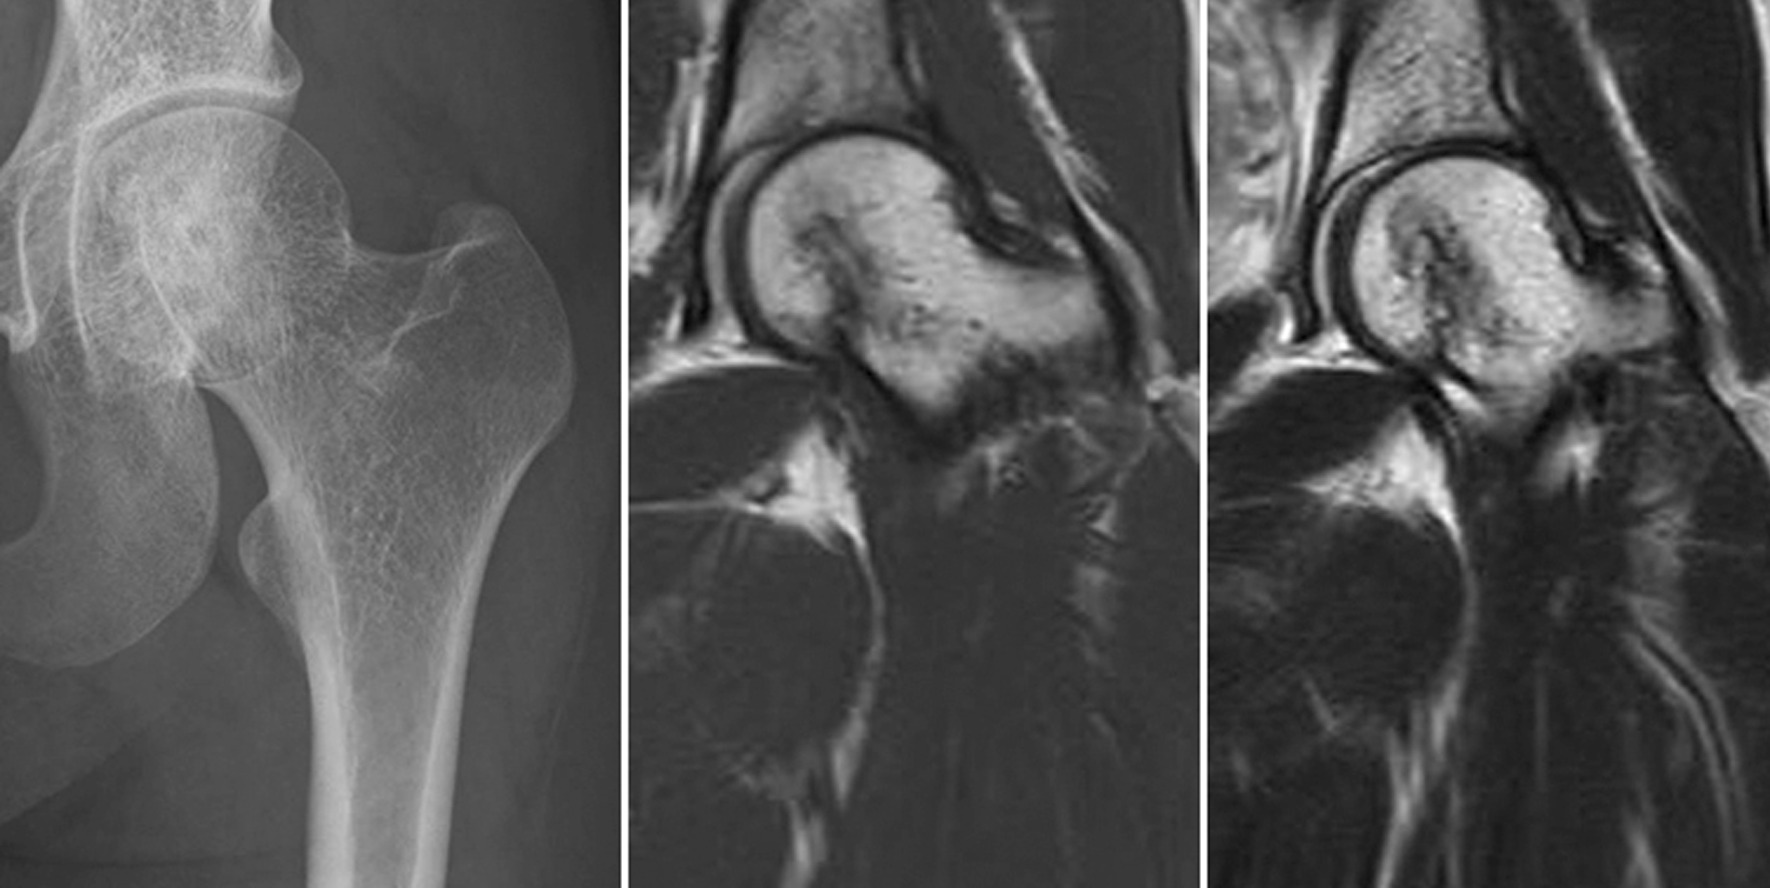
Imaging findings obtained 5 years postoperatively: Case 1. Plain X-ray image (left), T1- and T2-weighted (middle and right, respectively) MRI images demonstrating that there is no recurrence or avascular necrosis
